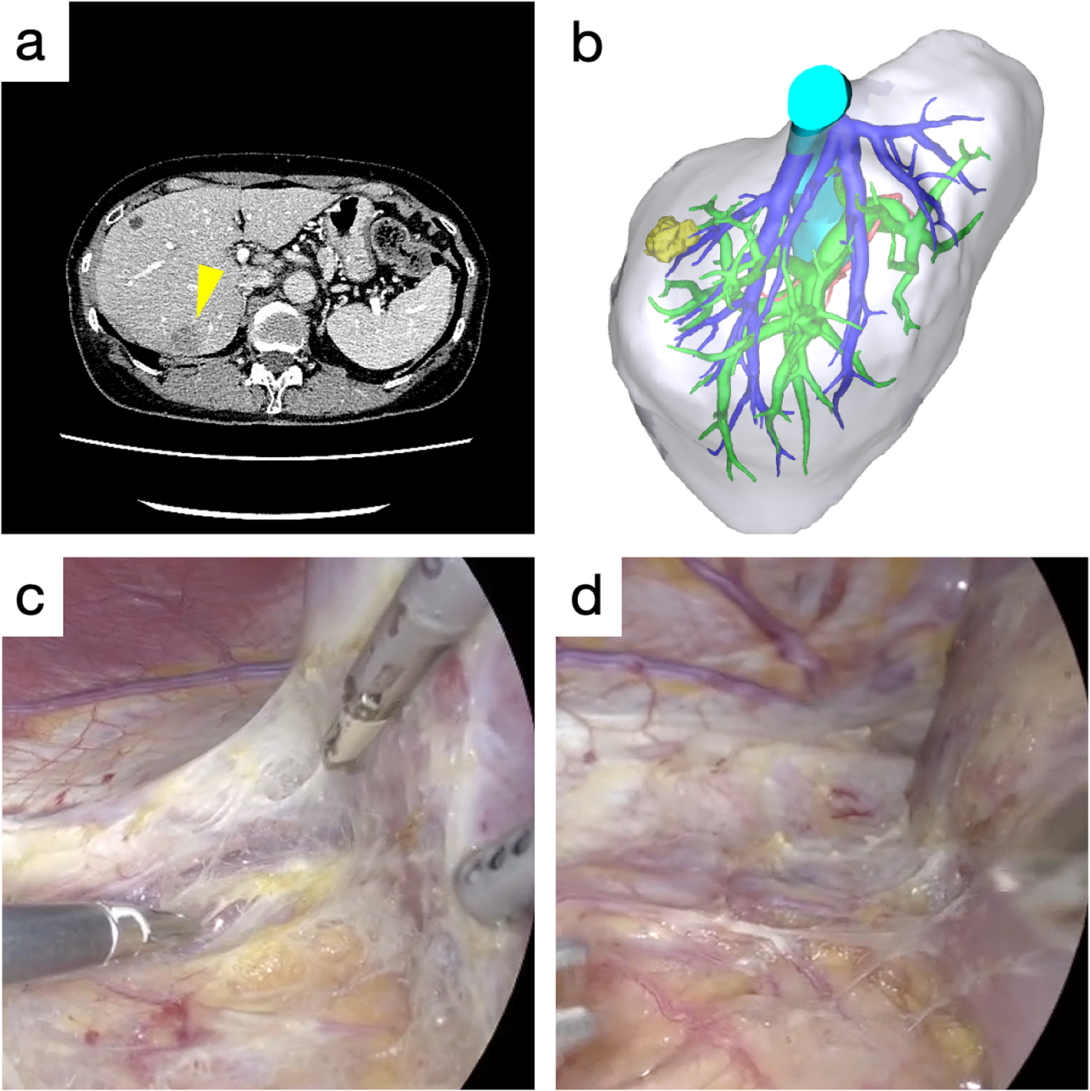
Preoperative and intraoperative images of the 64-year old woman with a colorectal cancer liver metastasis (Case 3). a Preoperative computed tomography image. The tumor is indicated by a yellow arrowhead. b Three-dimensional image reconstructed by SYNAPSE VINCENT. The tumor is indicated in yellow. c Laparoscopic partial hepatectomy was performed. The bare area dissected is the outer layer of the fused peritoneum. d The right liver upon dissection revealed a dense white connective tissue
